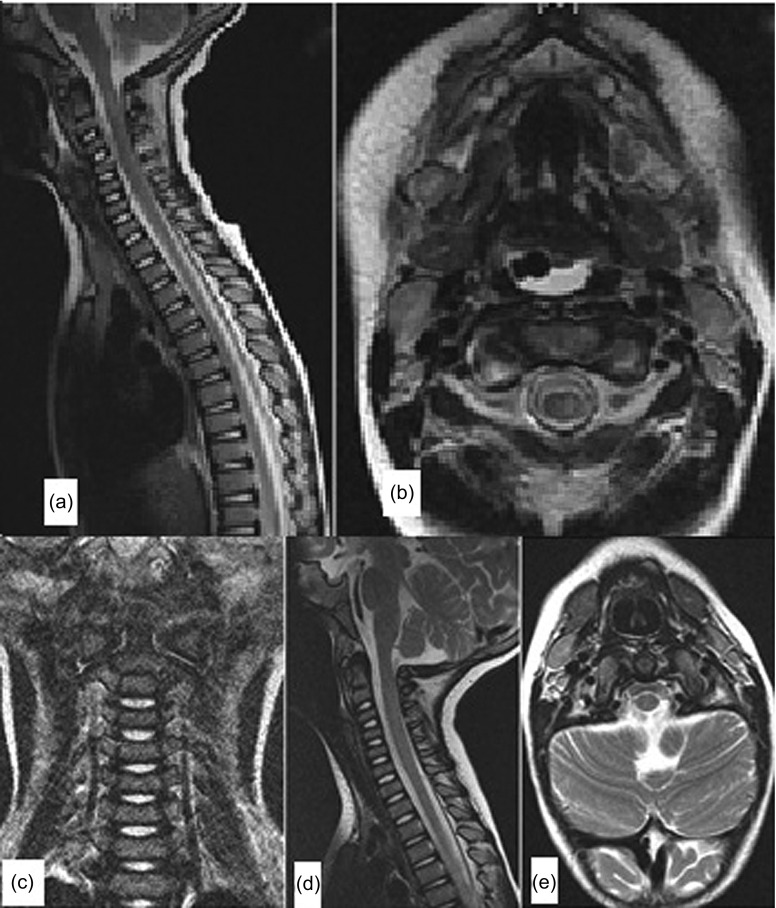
Case 1: (a, b) Pre-reduction MRI shows the disco-ligamentous injury at C3/4 level, and the spinal cord edema on the left side seen in axial cut. (c–e) Follow-up MRI shows the healing of the disco-ligamentous injury at C3/4 level, and complete subsidence of the spinal cord edema.
